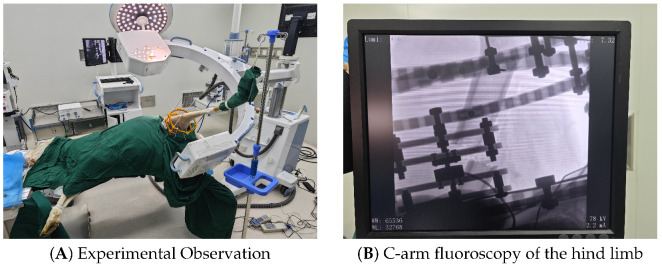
Radiographic validation. (A) Observation of the limb during the validation phase. (B) Following distraction protocol, confirming the generated distraction gap and the response of the soft tissue envelope.
